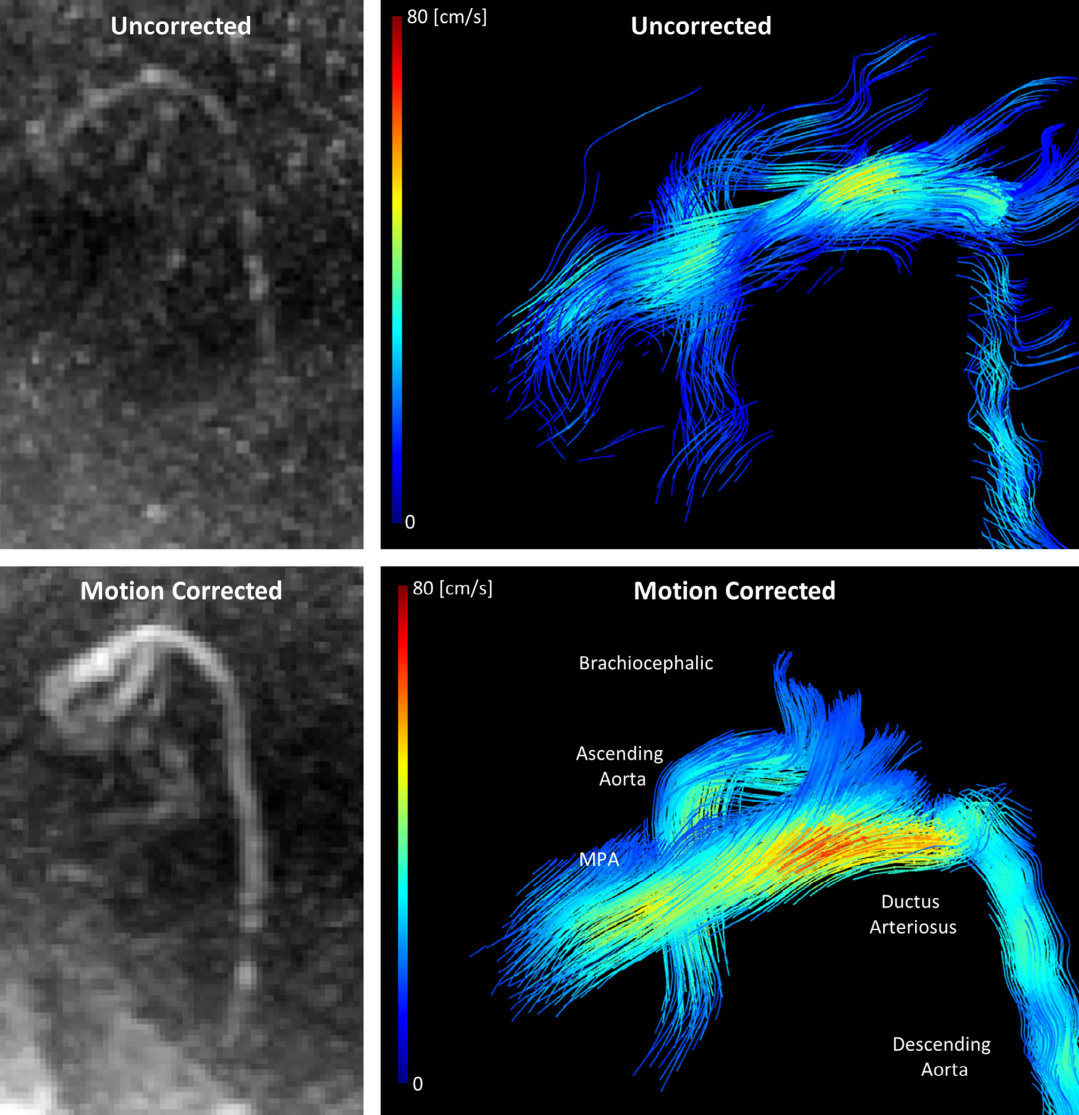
Comparison of PC‐MRA and velocity streamlines at peak systole for an unusable uncorrected scan (Acq. 19), using the segmentation of the motion‐corrected acquisition, and motion‐corrected 4D flow MRI data in a healthy participant, representing a “recovered” acquisition. MPA, main pulmonary artery.
